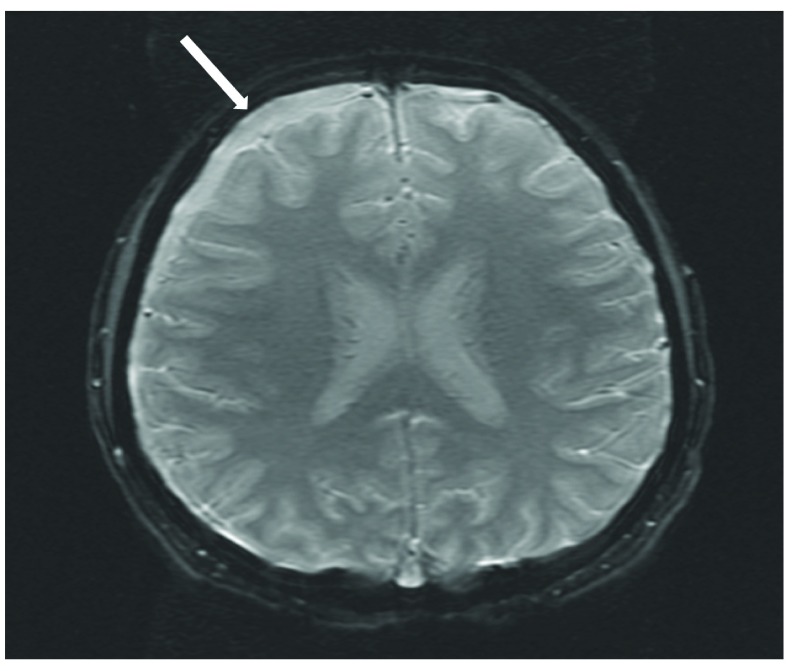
Axial susceptibility weighted image of MRI brain with an arrow indicating the extra-axial collection in right frontotemporal region.
